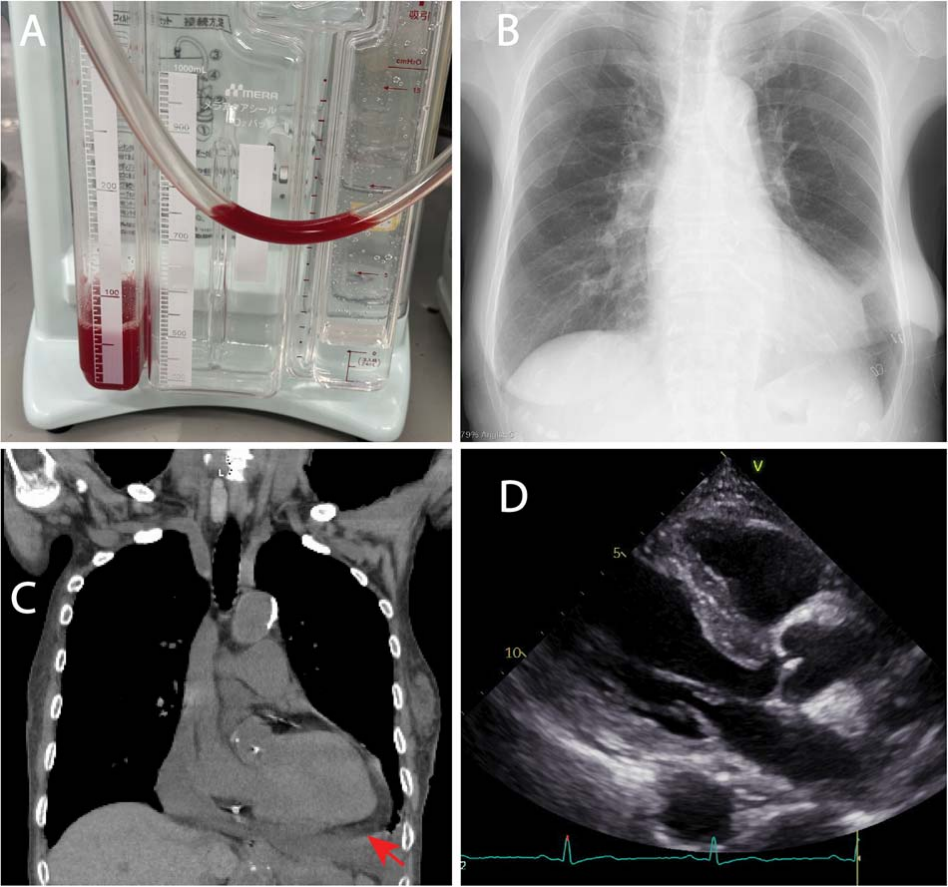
Postoperative images. (A) the pericardial effusion was slightly red. In total, 1200 ml of pericardial effusion was removed. (B) Postoperative chest X-ray. The cardiothoracic ratio was 0.45. (C) Postoperative computed tomography scan revealed little remaining cardiac effusion (arrow). (D) Postperative echocardiogram revealed little cardiac effusion.
